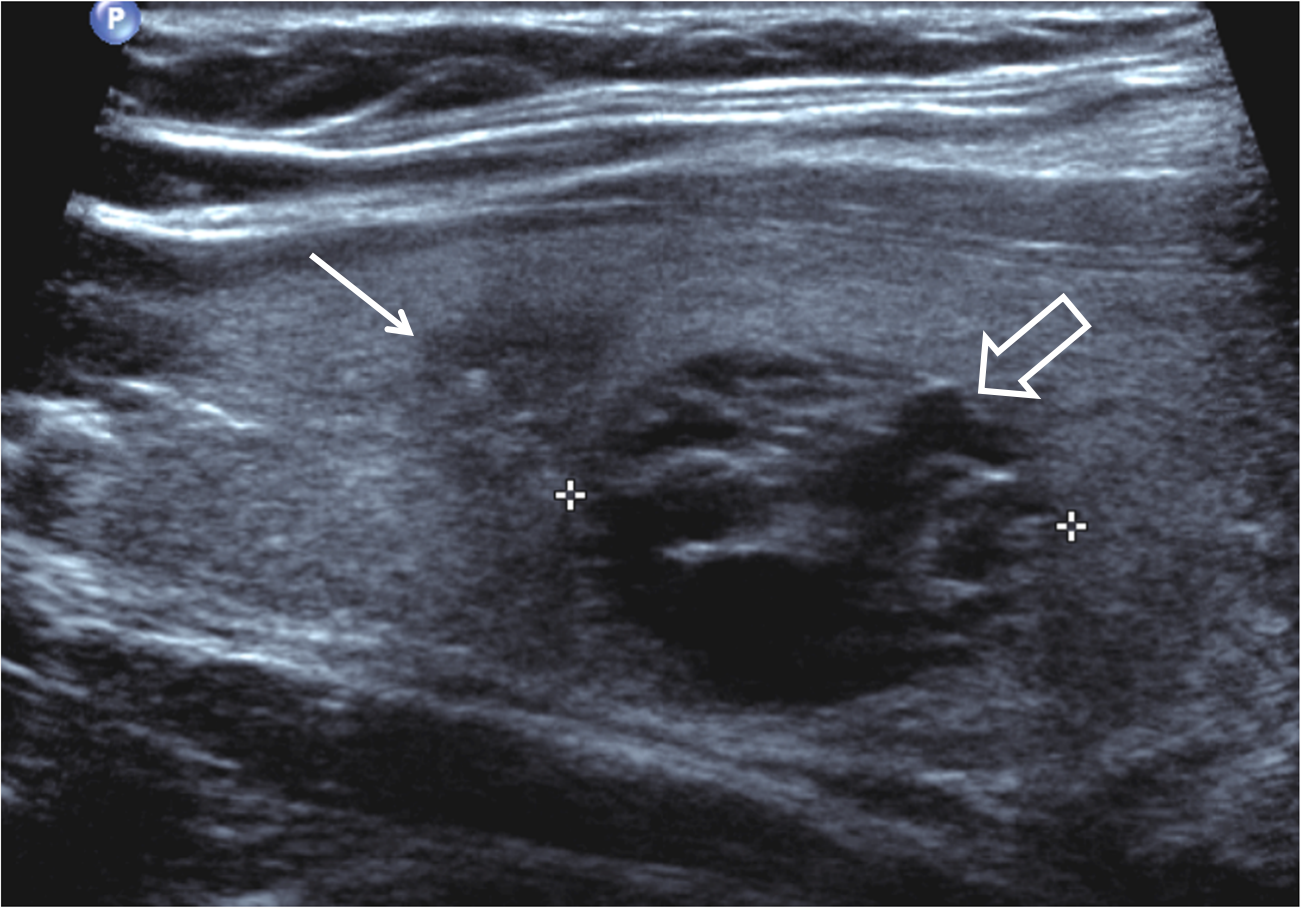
Longitudinal image of a 1.5-cm nodule (*block arrow*) detected incidentally on cervical spine MRI. This nodule is spongiform and appears confidently benign. However, a 0.6-cm ill-defined nodule alongside (*arrow*) is hypoechoic and contains microcalcification—FNA was performed from this nodule and confirmed papillary thyroid carcinoma
